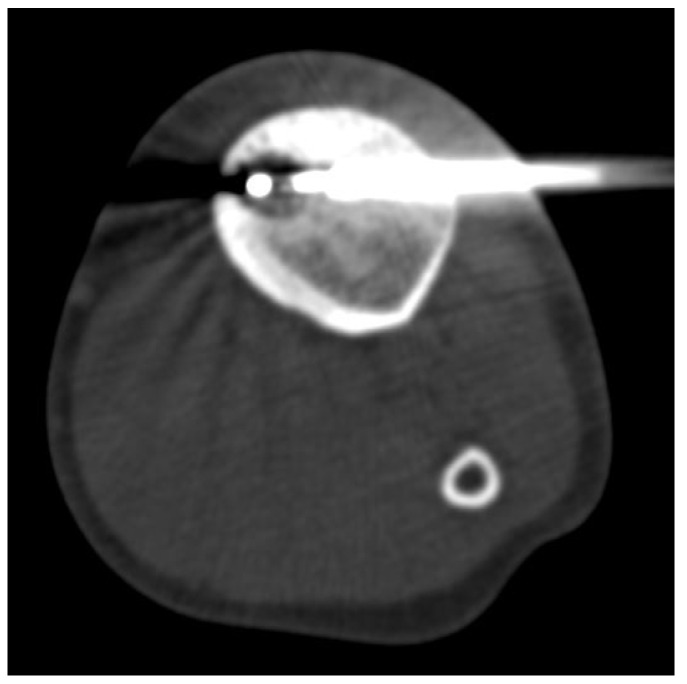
Cryoablation of tibial Osteoid Osteoma. CT image during the procedure showing the extent of the iceball’s extent and the distal tip of the cryoprobe at the center of the “nidus”. In this procedure, we performed a contralateral access to the “nidus” to avoid the thickened cortical portion.
